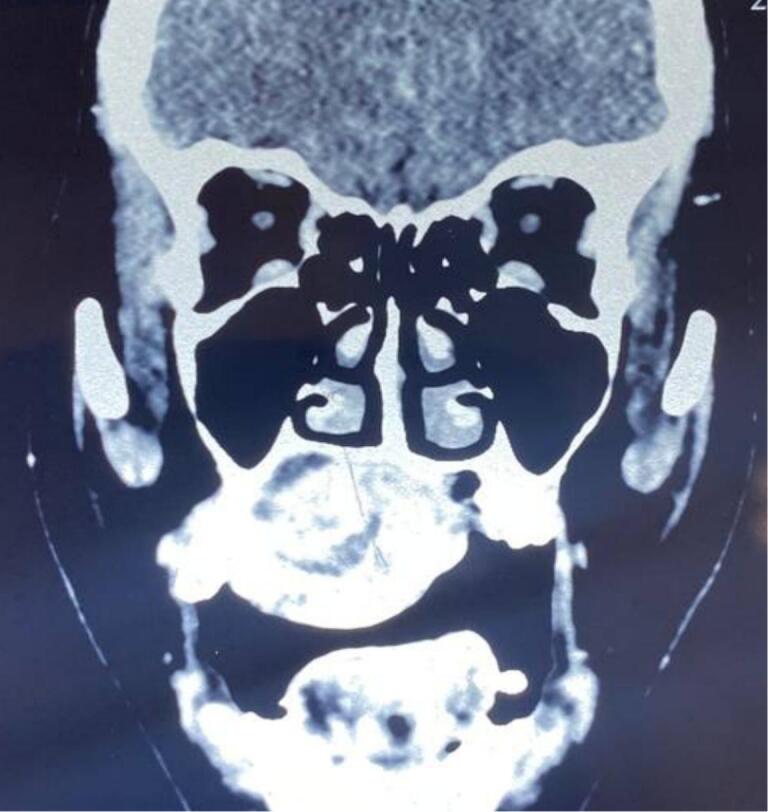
Coronal CT scan shoes expansive mass involving hard palate.
